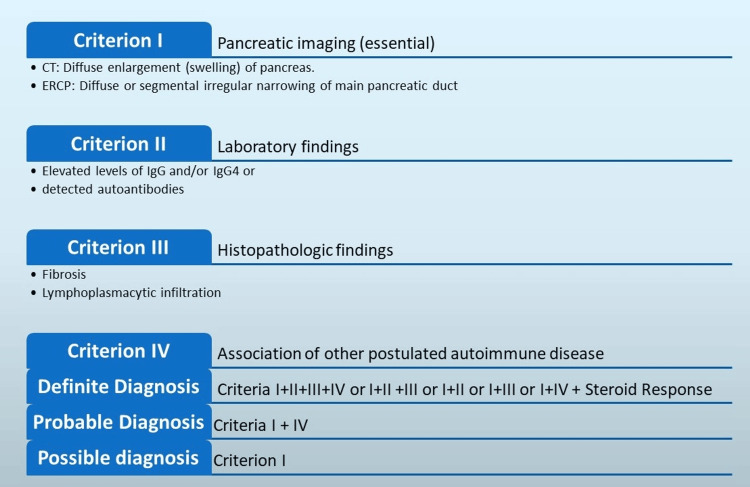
Diagnostic criteria for AIP diagnosis. CT: computed tomography, ERCP: endoscopic retrograde cholangiopancreatography, Ig: Immunoglobulin, AIP: autoimmune pancreatitis. Image credit: Adesola A. Agboola, Khalid H. Mohamed
